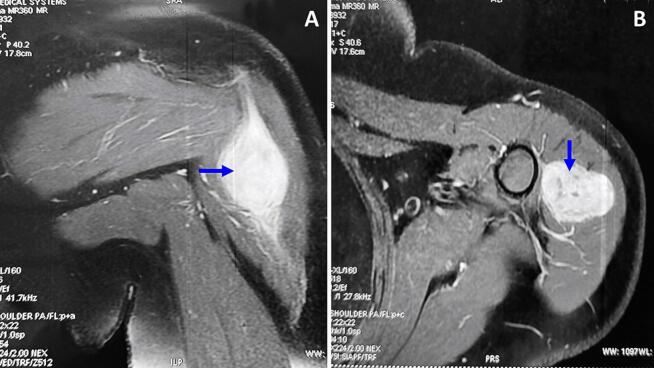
A: Coronal MRI showing the intramuscular tumor within the left deltoid muscle (Blue arrow). B: Axial MRI displaying the lobulated and indistinct lesion (Blue arrow) with a heterogeneous signal, appearing hyperintense on T2-weighted diffusion images. (For interpretation of the references to colour in this figure legend, the reader is referred to the web version of this article.)
